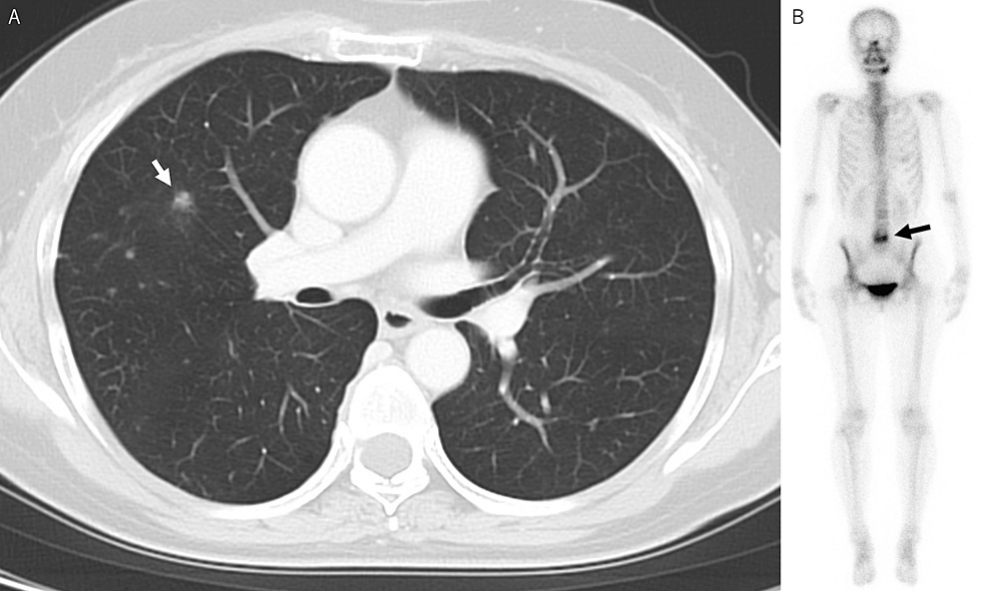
(A) Axial CT image of the chest at the initial examination. (B) Whole body bone scintigraphy at the initial examination. A. Metastatic lung tumor was seen in the right thoracic region (arrow). B. There was an accumulation in the L5 vertebral body, and a multisite metastatic bone tumor was not evident.
